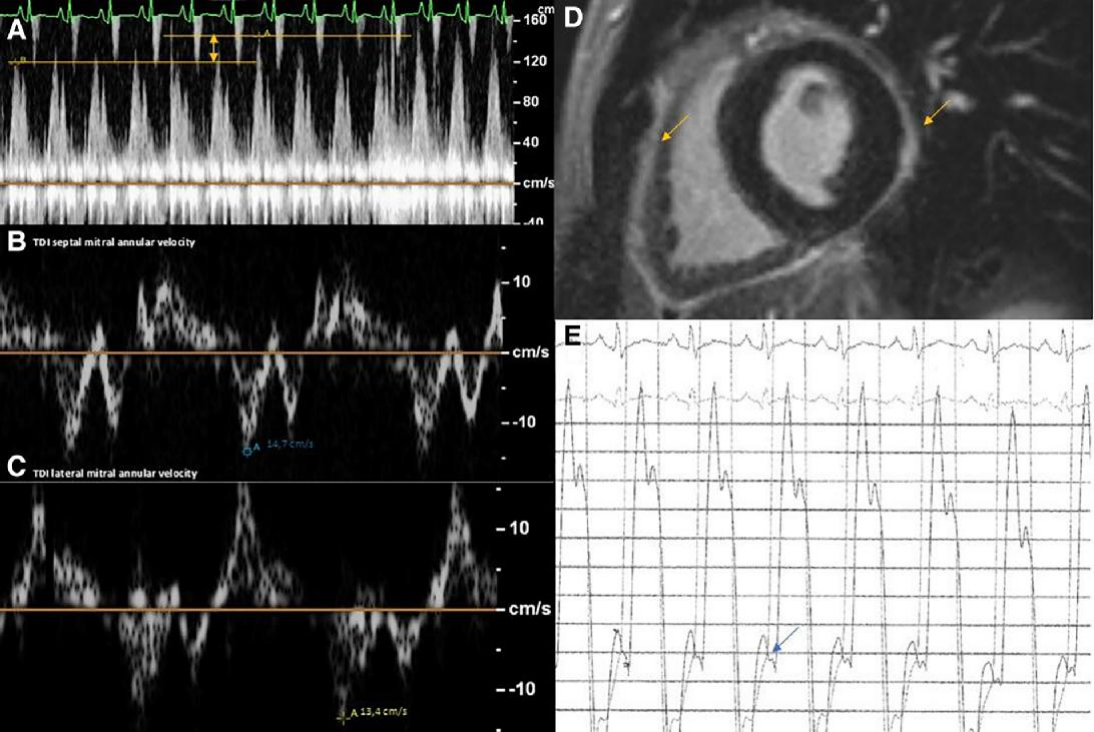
Echocardiography showing initial mitral flow respiratory variations (*A*) and normal mitral annular velocity with ‘annulus reversus’ (*B* and *C*). Cardiac magnetic resonance imaging (*D*) and cardiac catheterization (*E*) depicting increased pericardial thickness (orange arrows) and left ventricular and right ventricular diastolic pressure equalisation (arrows).
